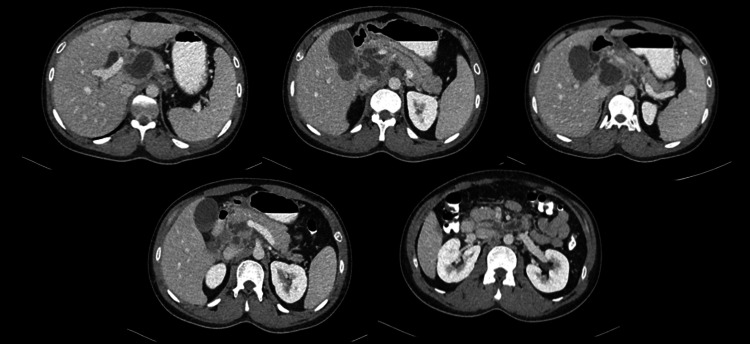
Axial images of contrast-enhanced CT of the abdomen A well-defined lobulated hypodense lesion with rim enhancement involving the pancreatic head, caudate lobe, and peripancreatic lymph nodes exerting mass effect seen as dilatation of the hepatic ducts, common bile duct, and pancreatic duct as well as compression of the portal vein and inferior vena cava with no thrombus formation CT: computed tomography
